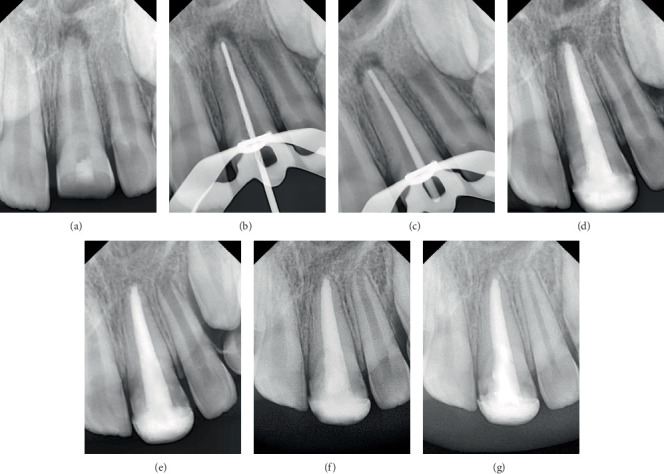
Case 2. (a) Preoperative periapical radiograph. (b) Working length radiograph. (c) Cone fit radiograph. (d) Postoperative radiograph. (e) A 6-month recall radiograph. (f) A 12-month recall radiograph. (g) A 24-month recall radiograph.
